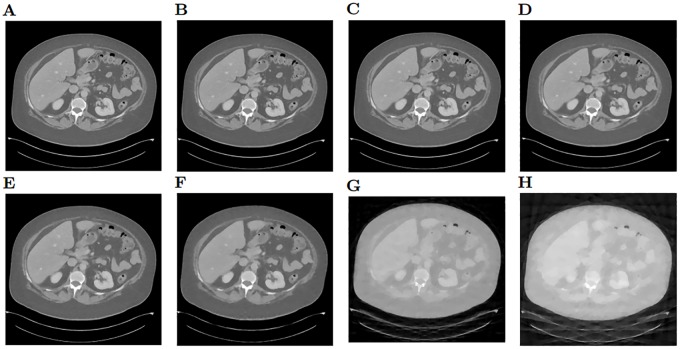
Reconstructions of an abdominal CT image. A: Reference CT image selected from dataset DeepLesion. B: Reconstruction using 180 projections. C: 150 projections. D: 120 projections. E: 90 projections. E: 60 projections. E: 45 projections. E: 30 projections.
